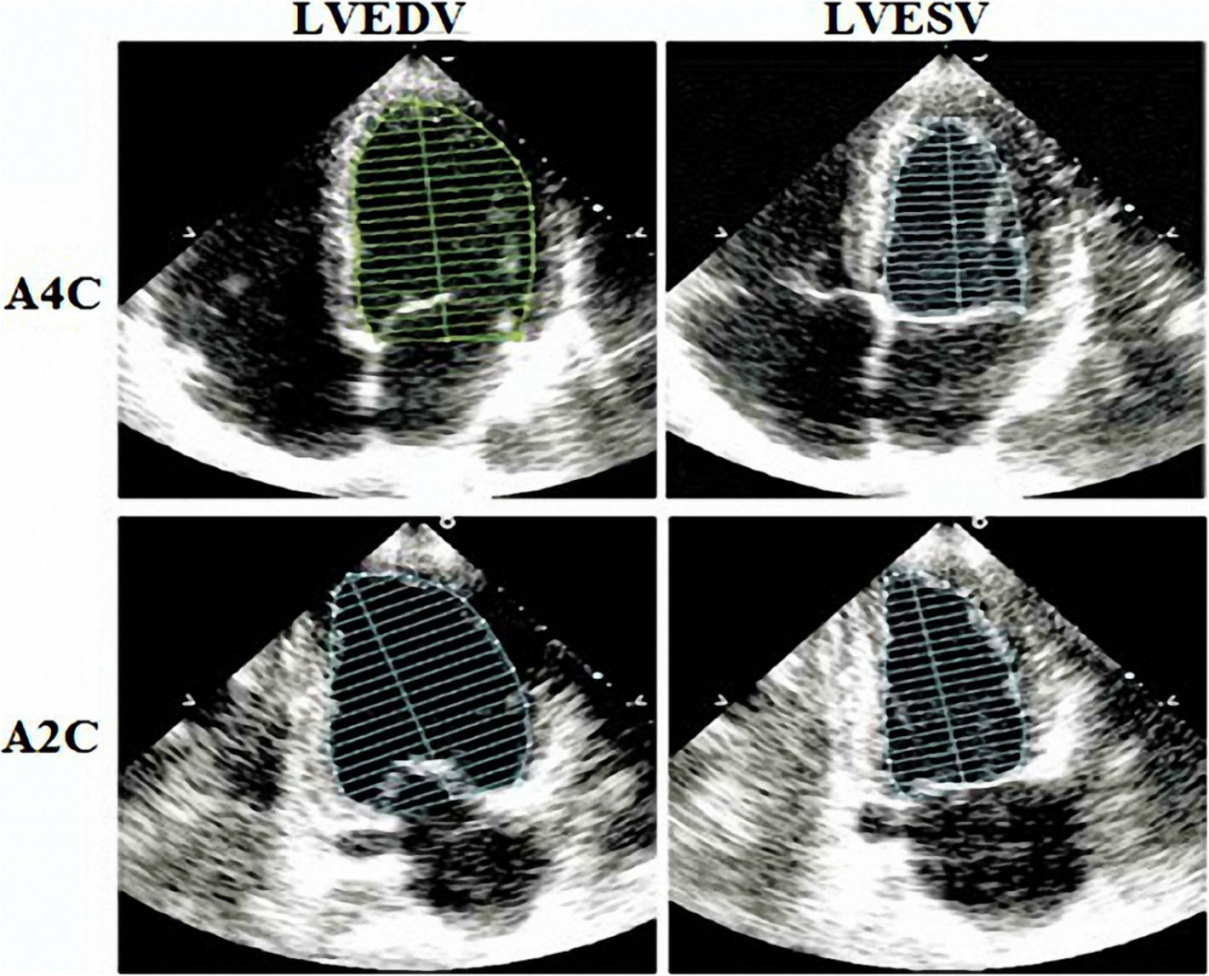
Method of calculation of biplane Simpson method. LVEDV: Left ventricular end-diastolic volume, LVESV: Left ventricular end-systolic volume, A4C: Apical 4-chamber view, A2C: Apical 2-chamber view
